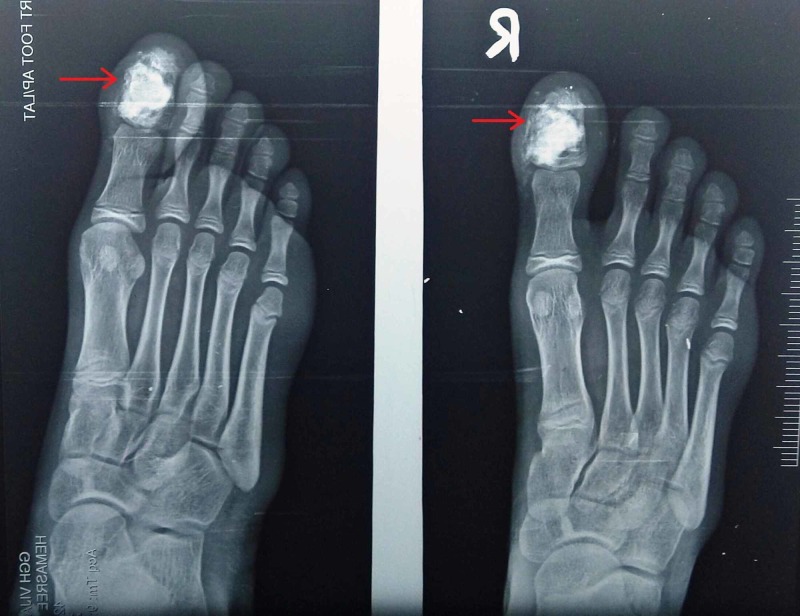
X-ray at six months follow-up The image shows incorporation of the graft
